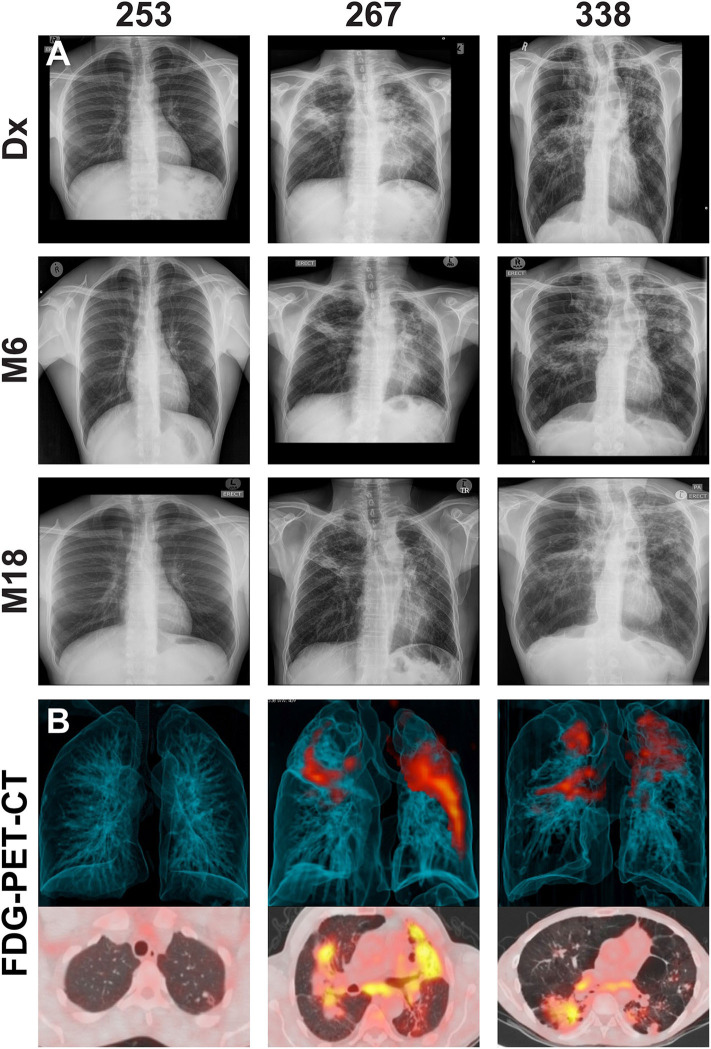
(A) Representative chest X-rays at diagnosis (Dx), month 6 (M6) and month 18 (M18) and (B) corresponding FDG PET-CT scan at M6 for cured patients with differentially culturable Mtb. The FDG PET-CT scans show 3-dimensional anterior (top panel) and transverse (lower panels) views.
